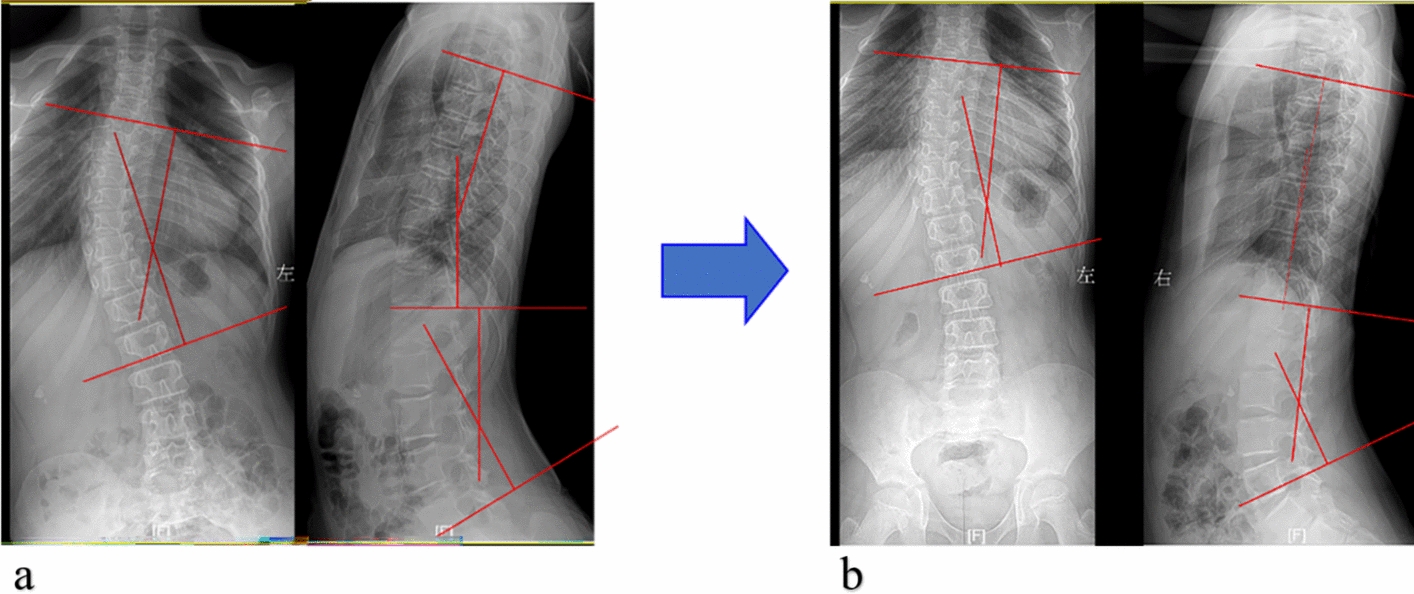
X-rays of patients before (a) and after treatment (b)
